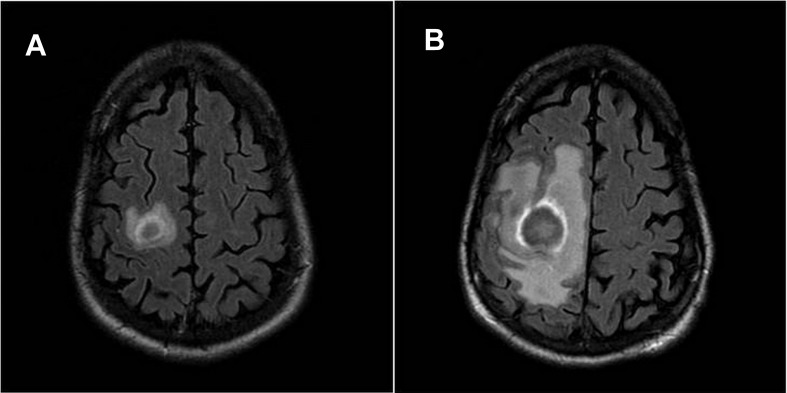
Brain MRI of patient 1 at presentation showed an ill-defined 1 cm right medial occipitotemporal gyrus mass and a 1.8×1.7×1.5 cm mass in the subcortical white matter of the right precentral gyrus with surrounding edema (
